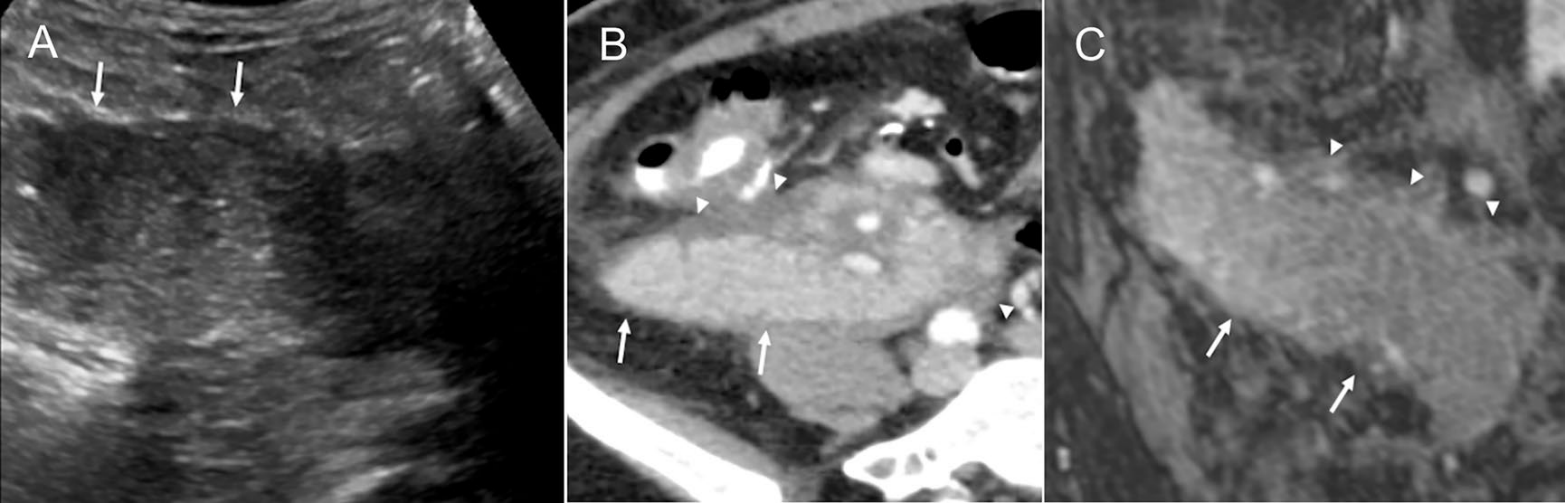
Acute graft pancreatitis. A 35-year-old female with a history of type 1 diabetes mellitus underwent pancreas transplantation alone and presented with persistently elevated blood glucose levels on postoperative day 12. Grayscale ultrasound (A) shows an enlarged pancreas graft with heterogeneous echotexture (arrow). Contrast-enhanced computed tomography (B) shows low-density acute inflammatory fluid (arrowhead) surrounding the pancreas graft (arrow), and magnetic resonance imaging (C) shows graft (arrow) enlargement with surrounding edematous changes (arrowhead). Rejection can have similar findings and is differentiated with biopsy
